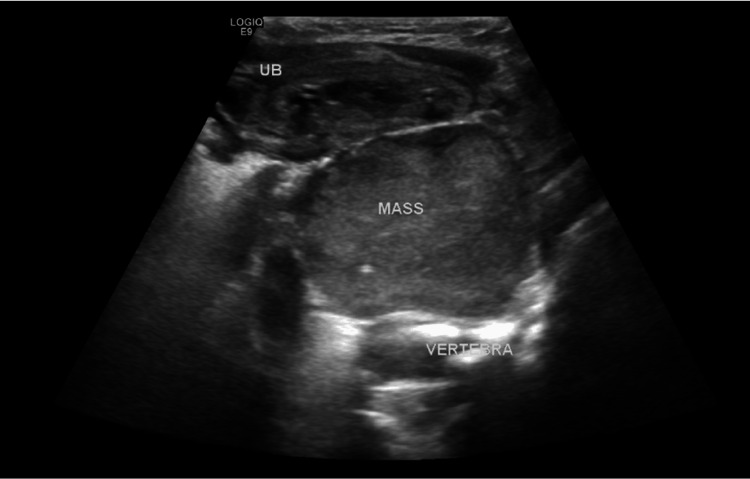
Mid-sagittal pelvic ultrasound scan with a large soft tissue mass (labeled) in the pelvis anterior to the vertebrae and posterior to the urinary bladder.
